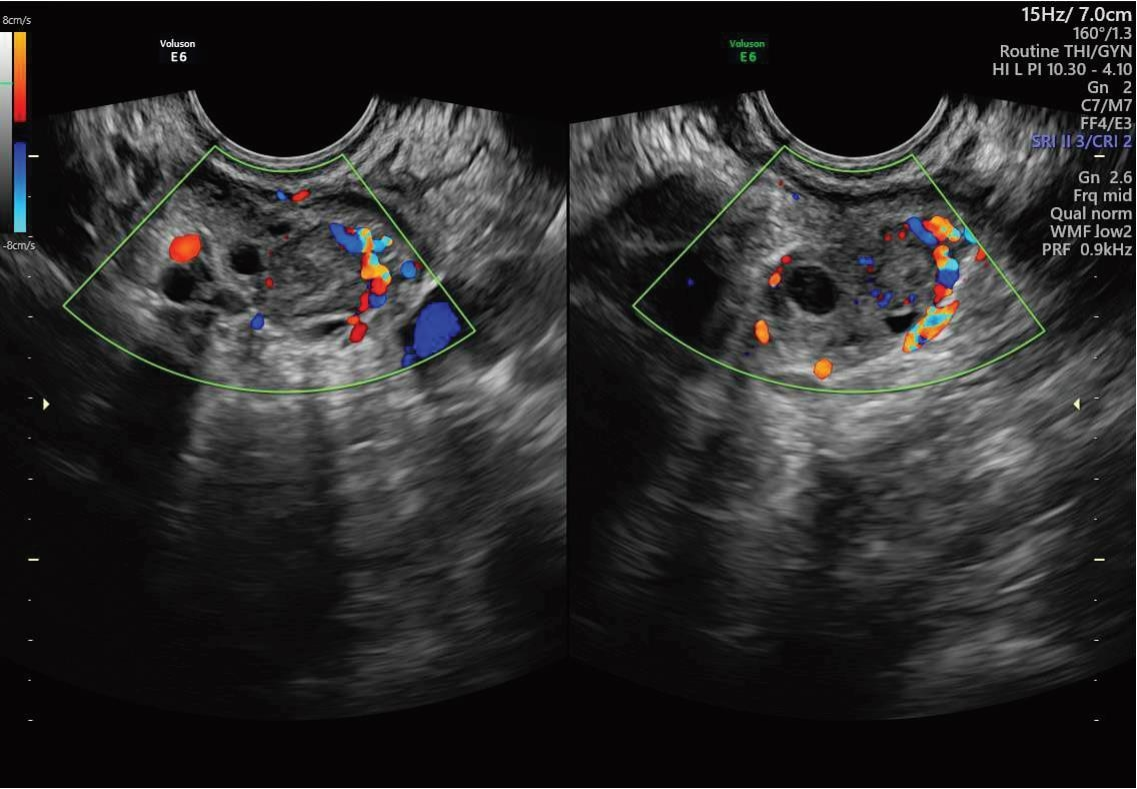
Transvaginal ultrasound at admission showing a corpus luteum on the left ovary.
